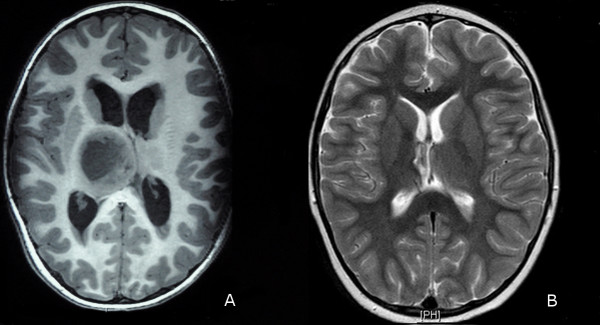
MRI of pediatric glioma. (A) MRI shows a large right thalamic tumor compressing the third ventricle. (B) MRI control 9 months later.
